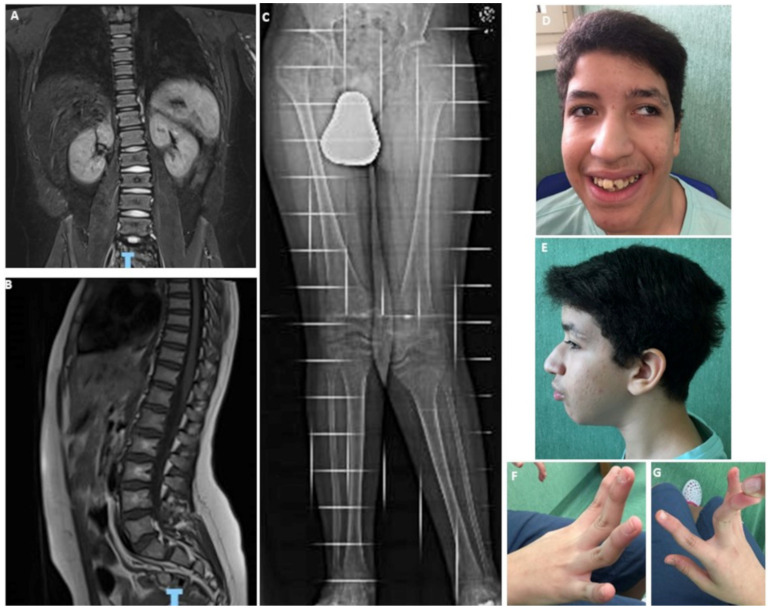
(A,B) MRI of lumbosacral spine at 13 years. Note the altered morphology of the vertebral bodies, which have a reduced height secondary to non-recent vertebral compression fractures, more marked in L5. The vertebral canal has a regular diameter. (C) X-ray of lower limbs showing right hip subluxation and a severely valgus left knee. (D–G) Physical examination of the patient at 13 years old, showing divergent strabismus, open mouth appearance, large and malpositioned teeth, hypermobility of distal interphalangeal joints.
